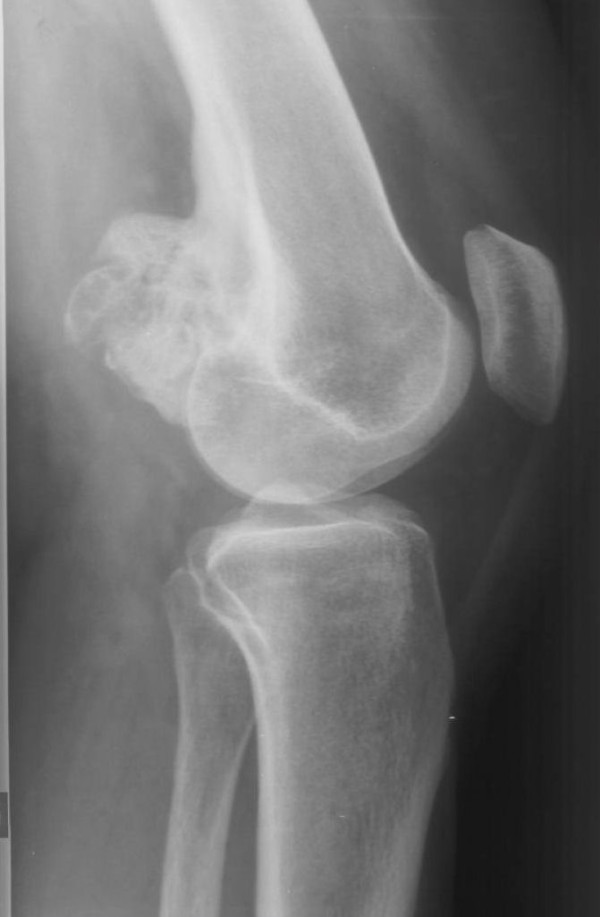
Case 2 - lateral view: Radiographs exhibit a lobulated ossified mass attached with a broad base to the postero-lateral surface of the left distal femur with no radiolucent cleft.
